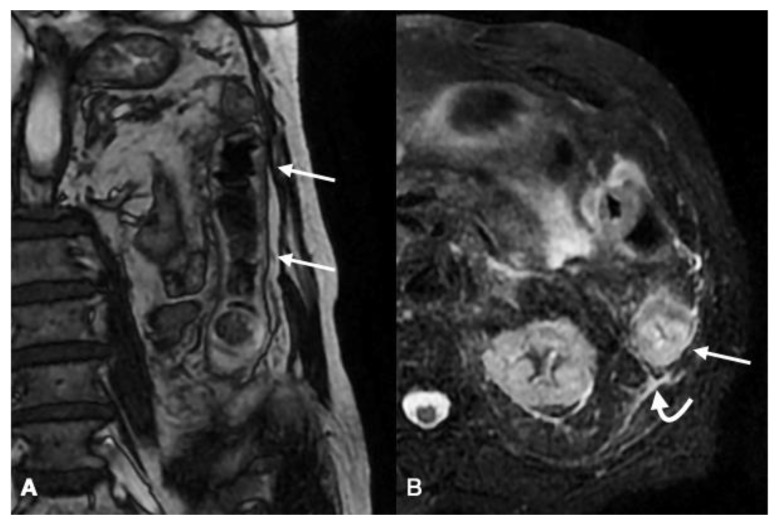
Follow-up MRI in an 80-year-old female with ischemic colitis, (A) T2W FIESTA sequence on the coronal plane and (B) T2W FatSat sequence on axial plane showing the continuous involvement of the descending colon ((A), arrows), characterized by oedematous colonic wall thickening ((B), arrow) with pericolic fluid ((B), curved arrow).
